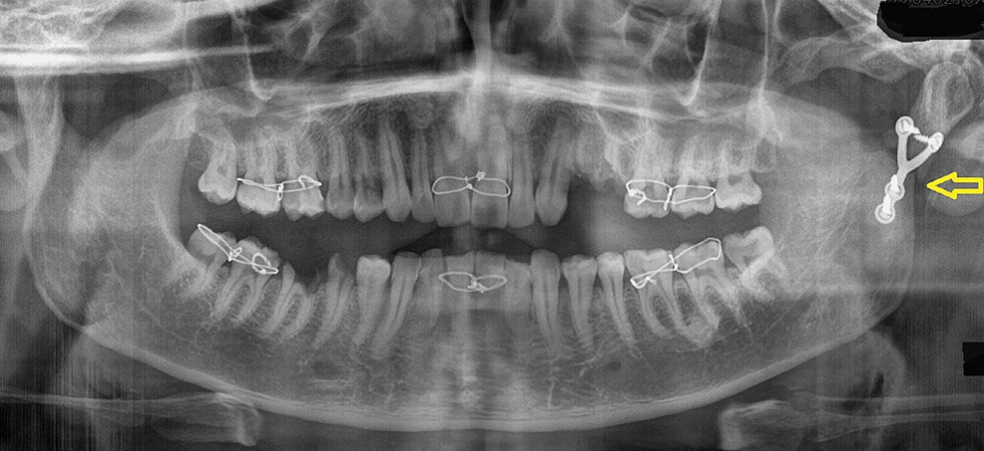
Postoperative OPG image OPG: Orthopantomogram Postoperative OPG showing good wound healing (arrow)
